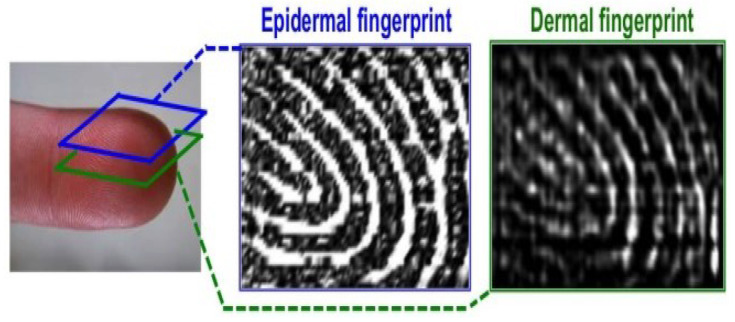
Ultrasonic finger images acquired from epidermal and dermal layers. Reprinted with permission from Ref. [33] 2016, IEEE.
